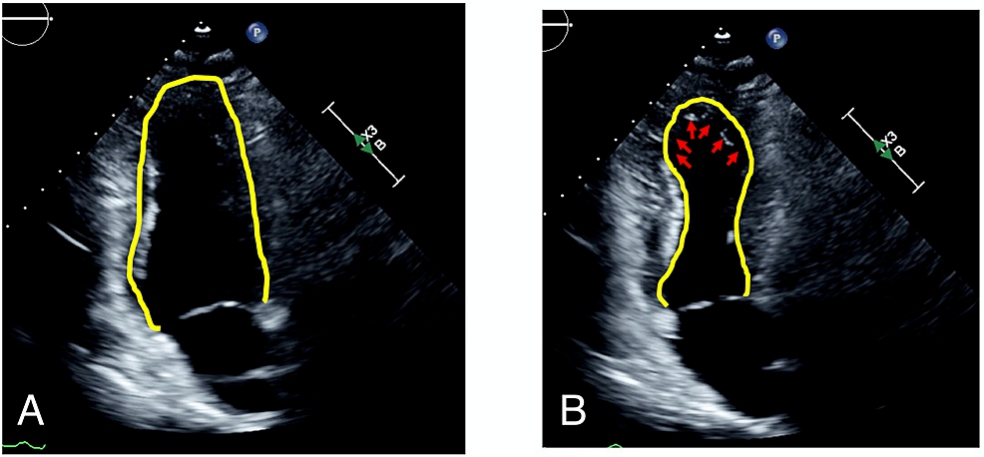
Transthoracic echocardiogram Apical 2 chamber view during diastole (A) and systole (B). Note apical anterior and apical inferior wall motion abnormalities.
